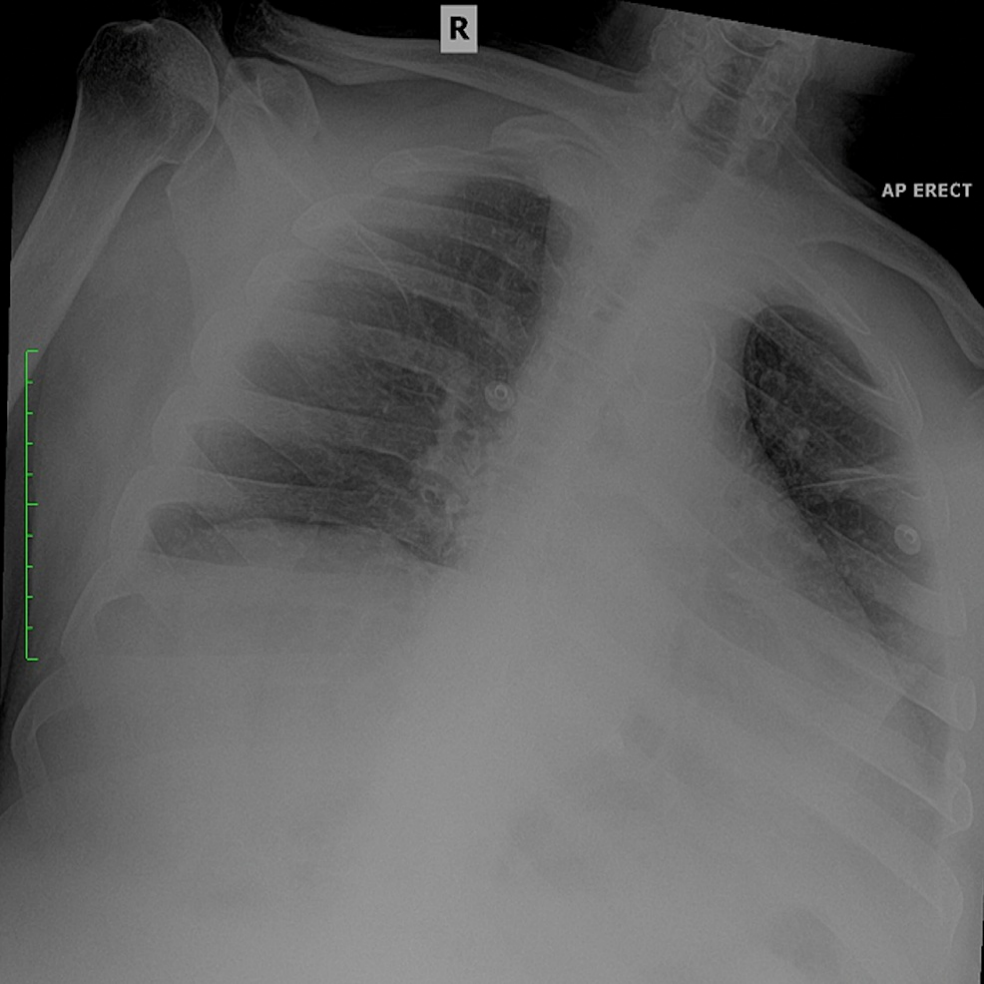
Chest radiograph on admission demonstrating bilateral basal lung infiltrates.
